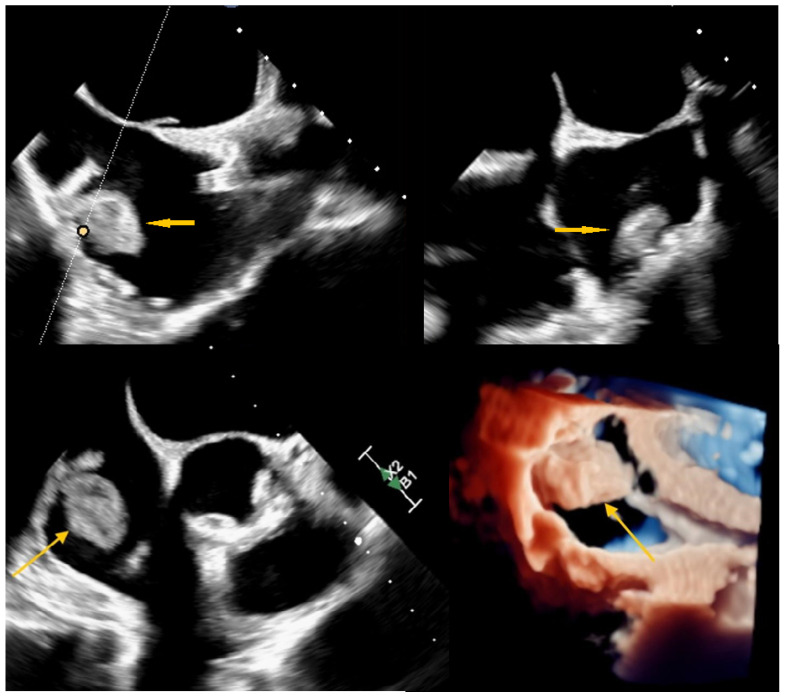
Four-chamber transoesophageal echocardiography (TEE) was subsequently performed to characterise the intracardiac mass further (yellow arrow). The study did not reveal any connection between the lesion and the vascular access port. Based on its echogenic appearance, location, and mobility, the RA mass was considered to be most consistent with a cardiac myxoma. The arrow indicates the balloting mass within the RA in 3D TEE (yellow arrow). The patient was evaluated within the framework of a multidisciplinary heart team and subsequently referred to the cardiac surgery department. A video recording can be found in Supplement S4.
